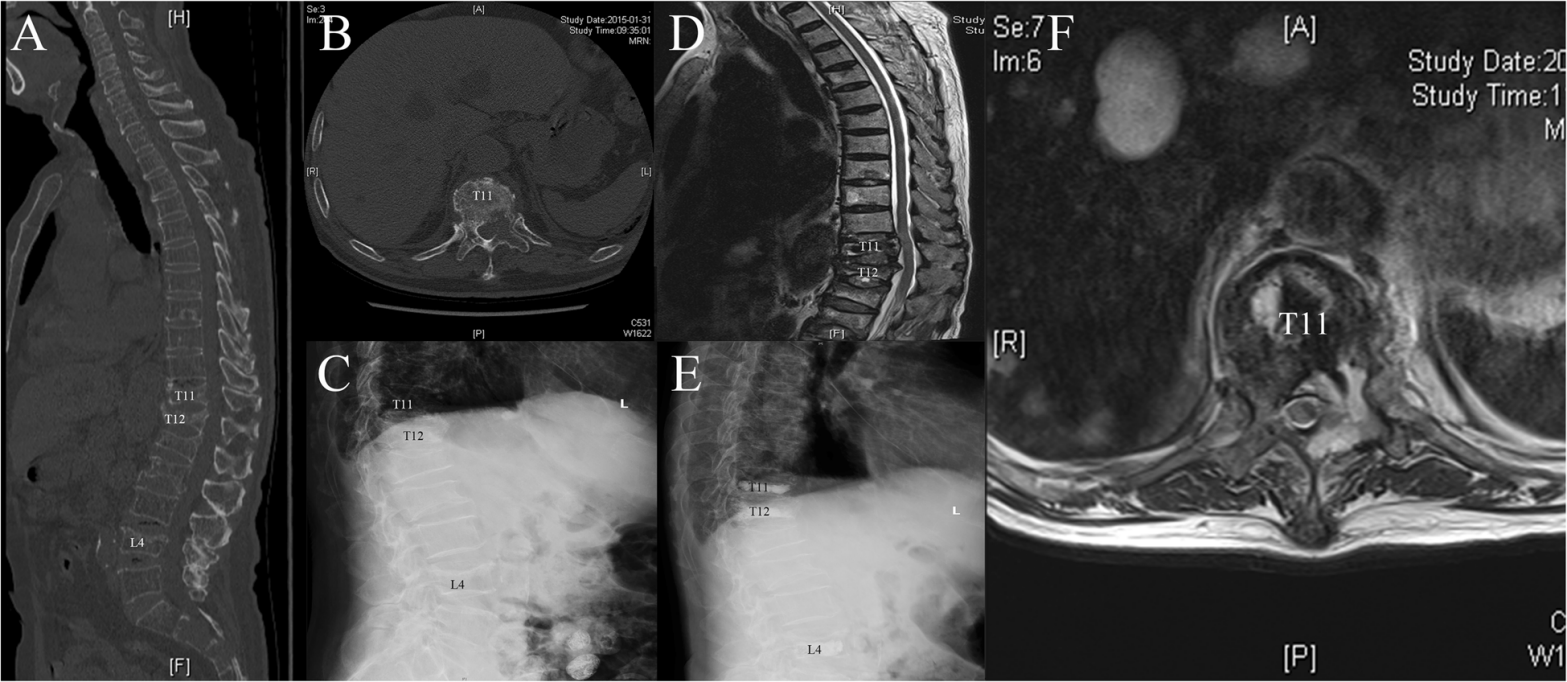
Images from one patient who developed lower extremity paralysis after surgery. a–c Patient with multiple myeloma with a pathological fracture of T12/T11/L4. CT scan showed that T11/12/L4 vertebral body osteolytic destruction, no spinal canal compression. e Postoperative X-ray plain film scan indicated that the bone cement were implanted successfully. d, f MRI revealed soft tissue formation in T11 vertebral body and left pedicle, and paraspinal and costal thoracic joints
